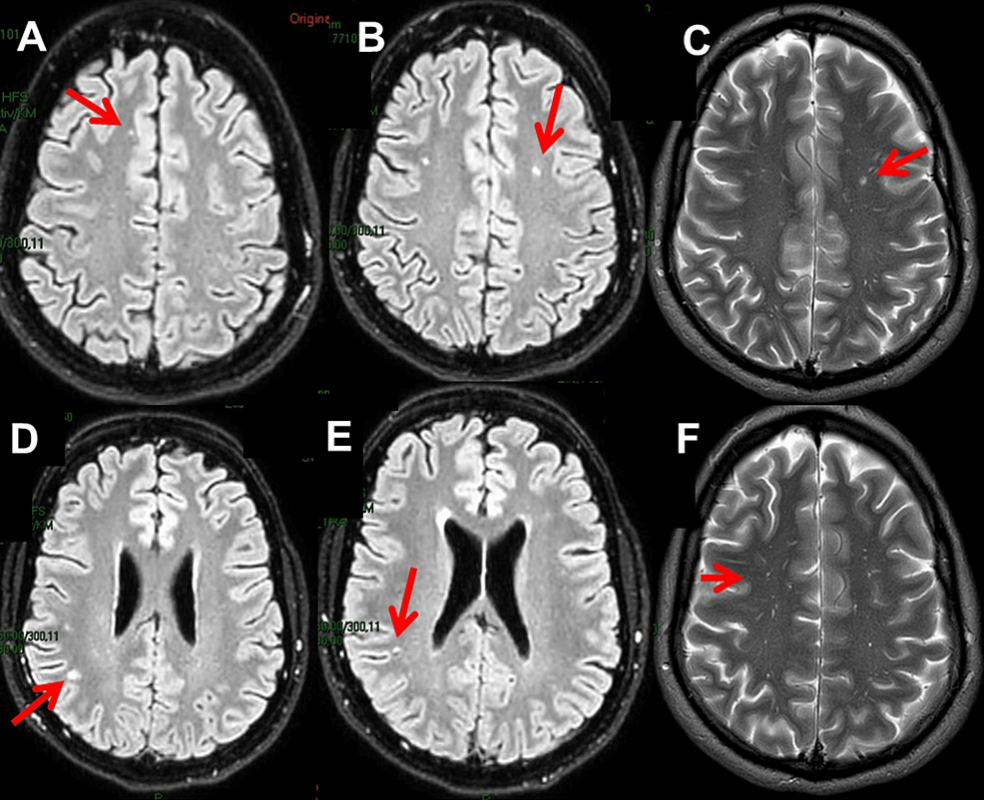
Cerebral MRI two months after the onset of LPCVS showing spot-like T2 (A, B, D, E) and FLAIR (C, F) hyperintensities in a frontotemporal distribution LPCVS: Long post-COVID vaccination syndrome, FLAIR: Fluid attenuated inversion recovery
